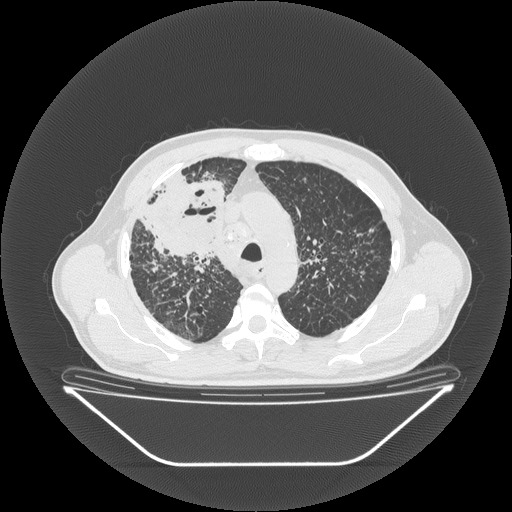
Preoperative thoracic CT scan upper right lobe suppuration
